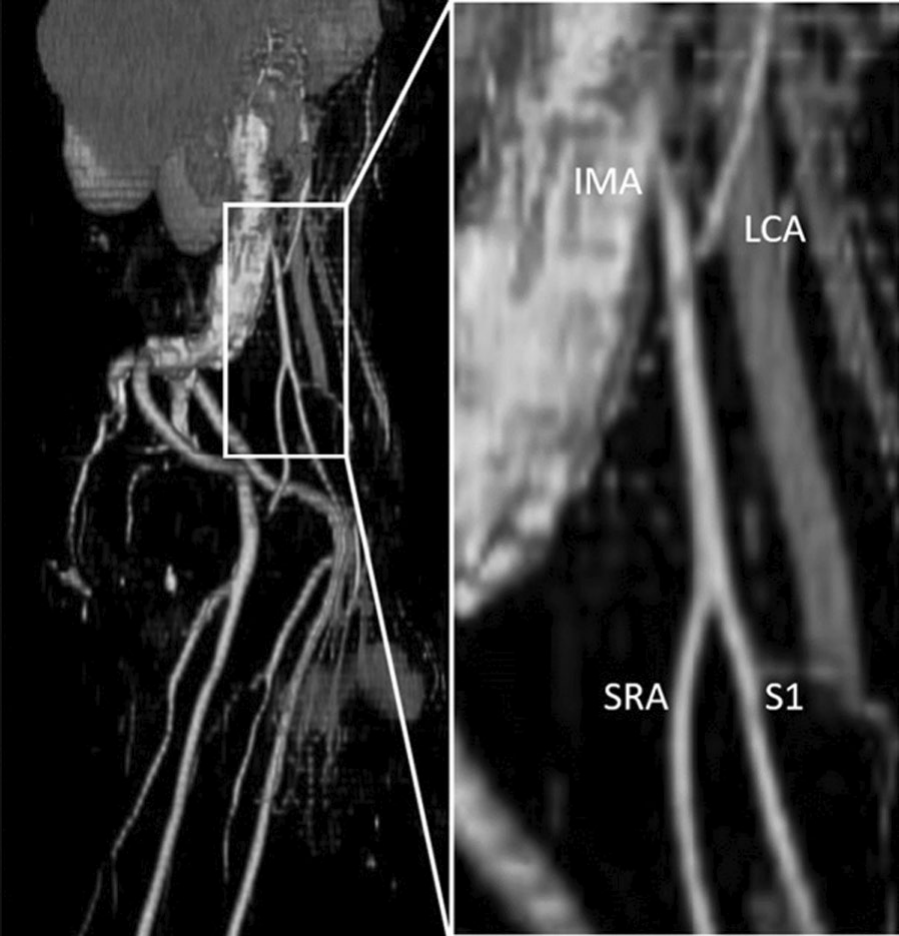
Reconstructed arteria image based on contrast-enhanced CT. Sigmoid artery (S1) proceeded the hernia sac after branching from IMA to the LCA and then to the SRA
